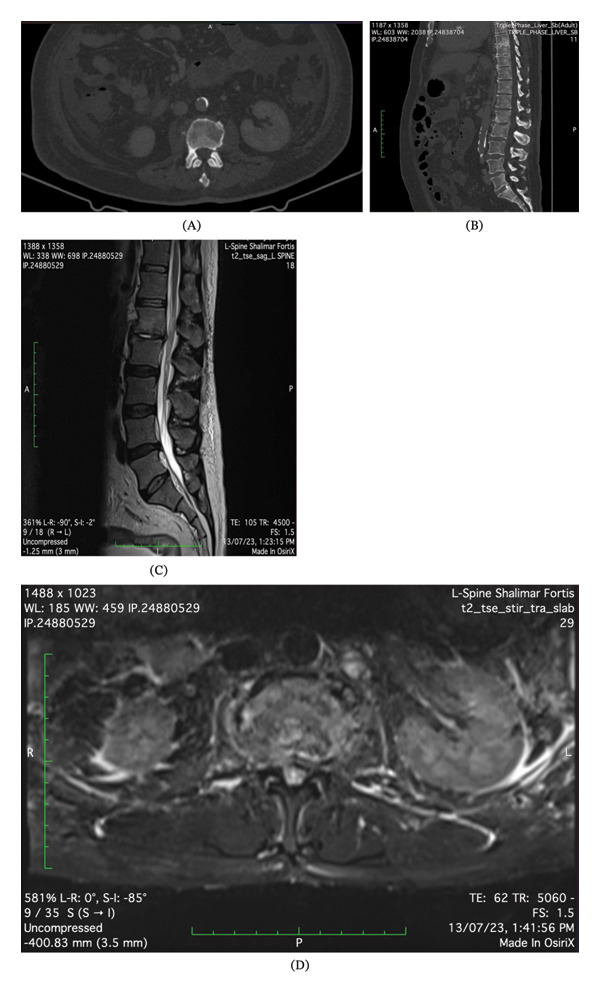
Initial CT and MRI images. (A, B) Noncontrast CT, axial and sagittal planes, demonstrating the involved level. (C) T2‐weighted sagittal MRI. (D) Axial contrast‐enhanced MRI showing disc and adjacent end plate involvement.
